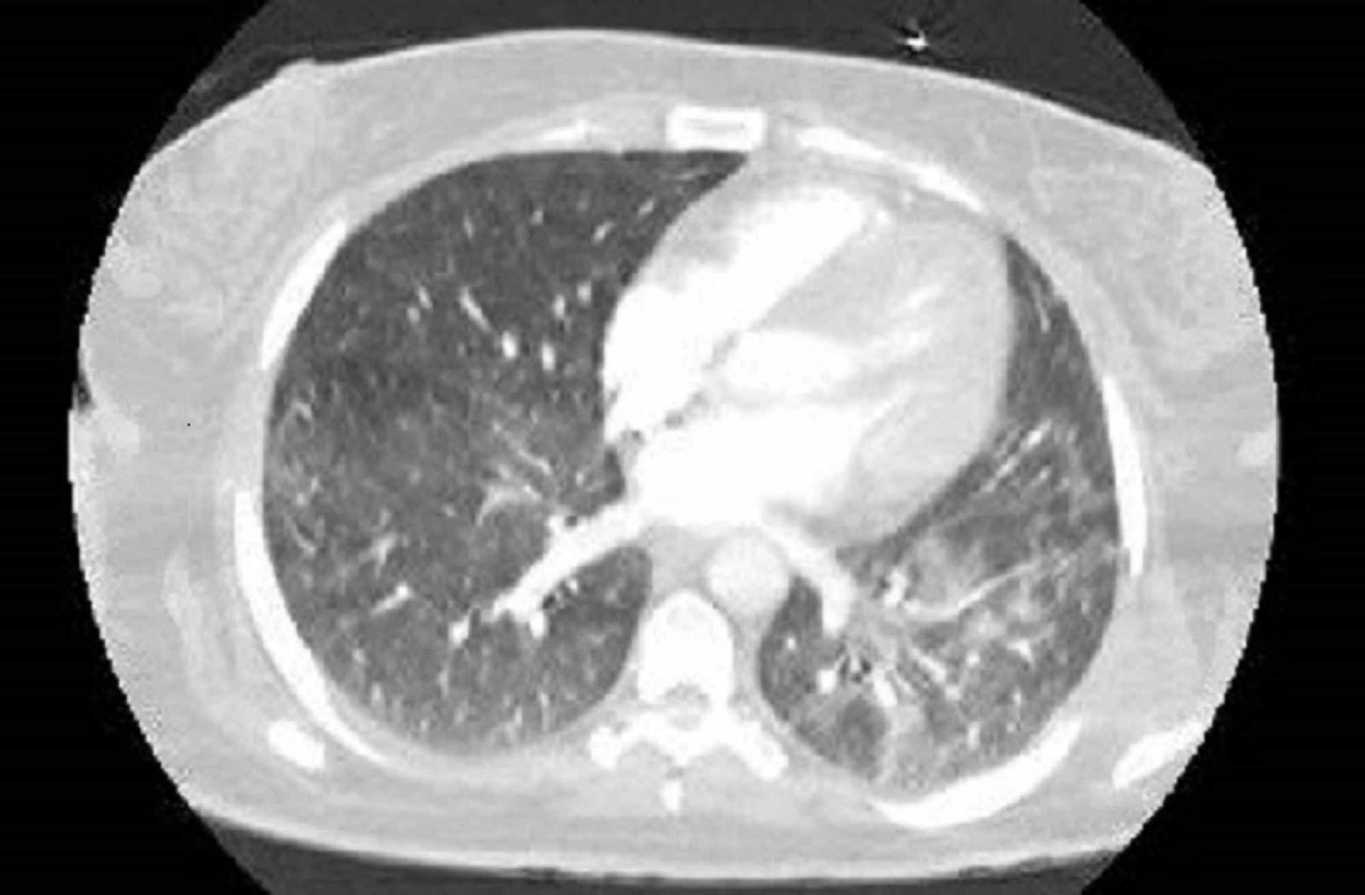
CT chest showing interstitial infiltrates
